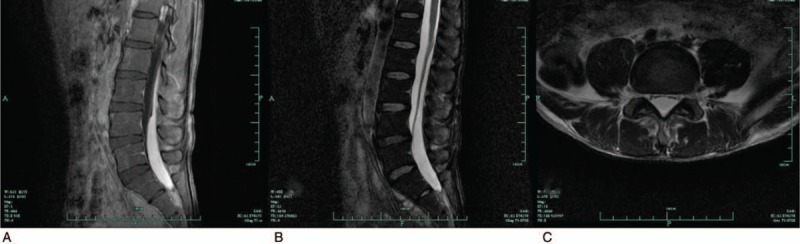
Repeated MRI scan of the lumbar spine at our center 15 days after onset. The size of subdural hematoma increased from L4 to S1 levels in the sagittal views, presenting as hyperintense signals on both T1-weighted and fat-suppressed T2-weighted sequences (A, B), and the cauda equina was dorsally compressed in the axial views (C). MRI = magnetic resonance imaging.
